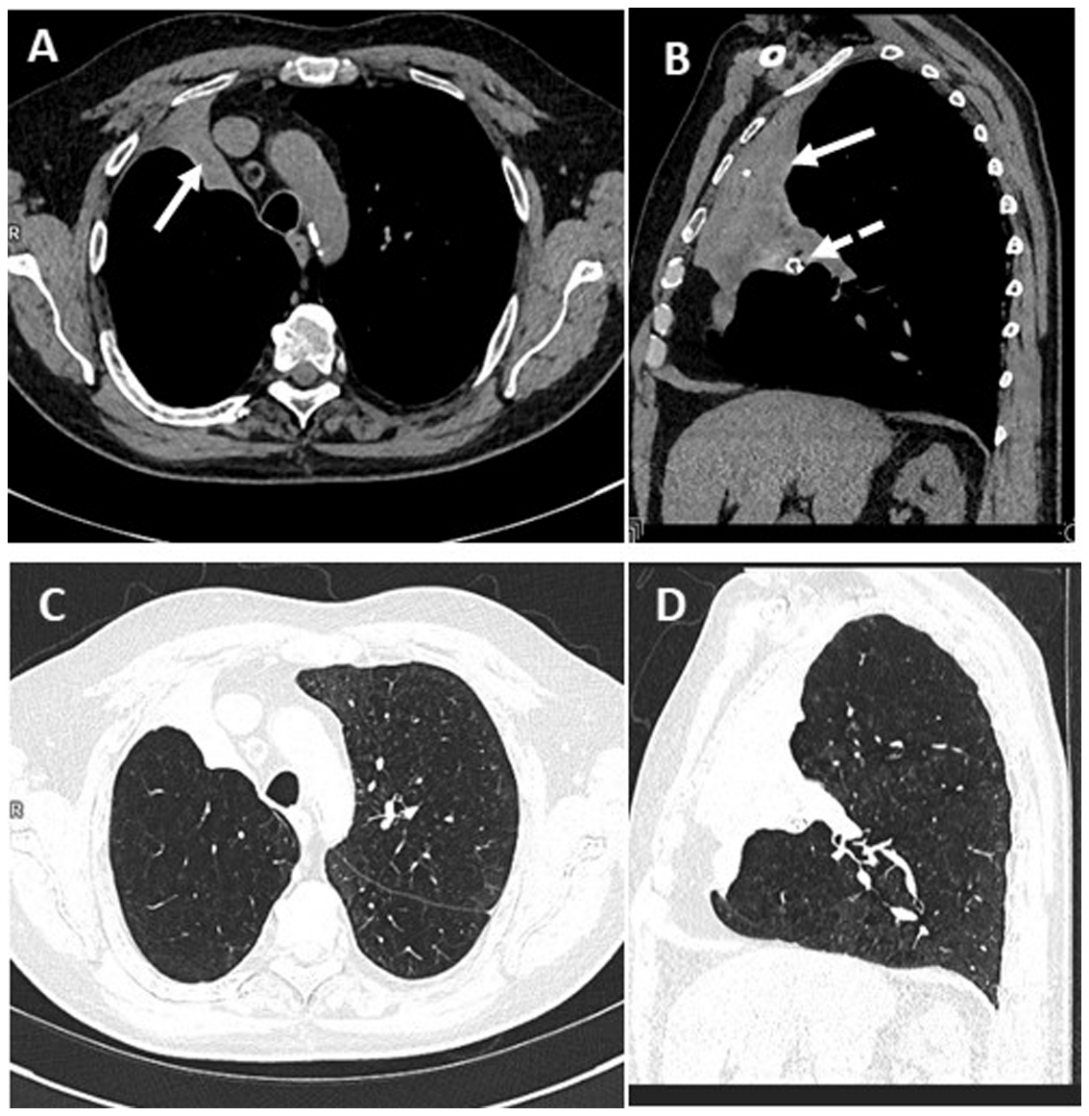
Axial and sagittal CT slices passing through the atelectasis, in mediastinal (A,B) and pulmonary (C,D) windows. The white arrow shows the atelectasis; the white dashed arrow shows a Zephyr endobronchial valve.
